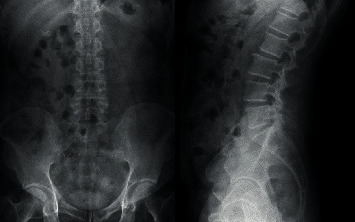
Postoperative X-ray films at the 1-year follow-up. Increased instability is not apparent in either the anteroposterior or the lateral view.
